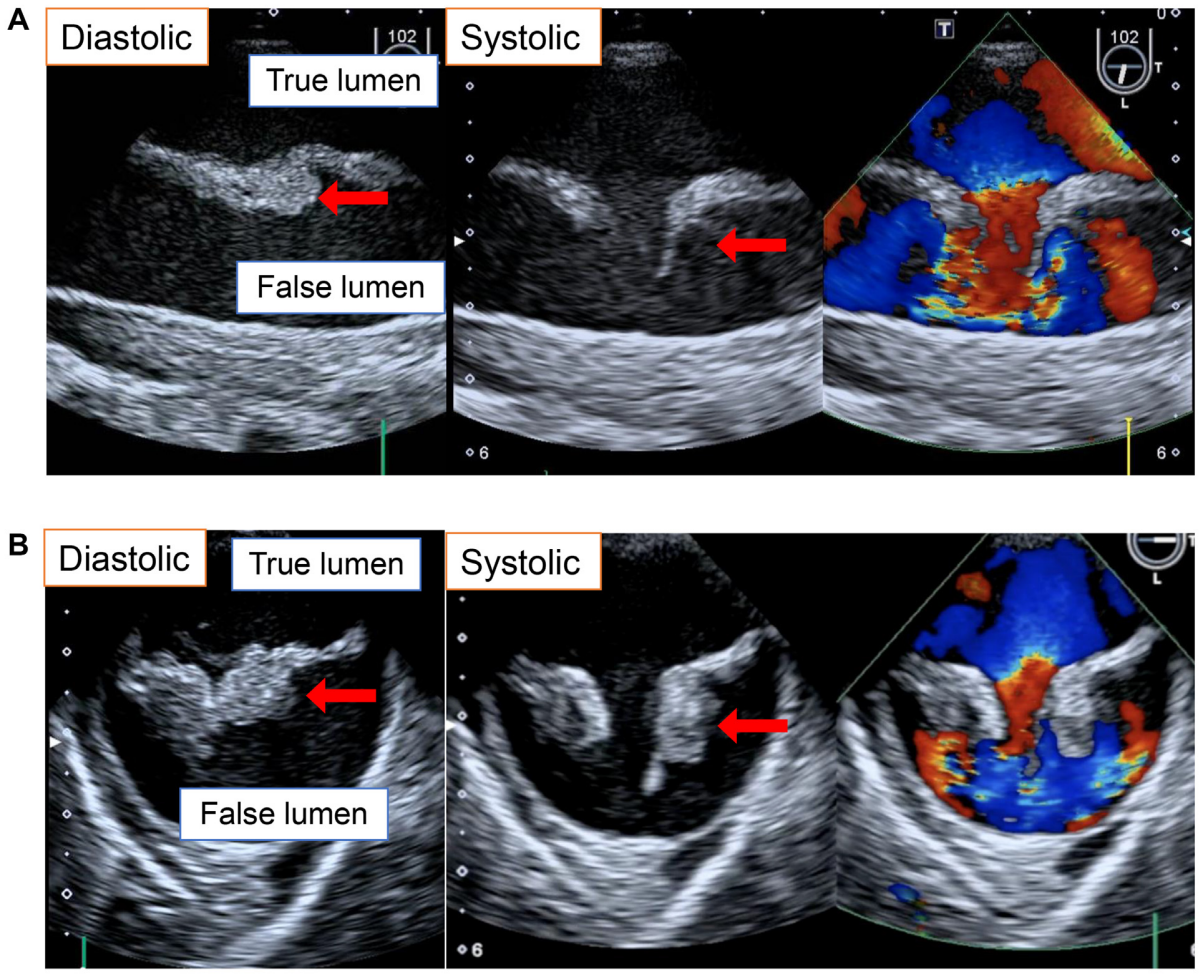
Echocardiographic images of proximal descending aorta with and without color Doppler in long-axis view (A) and short-axis view (B).
